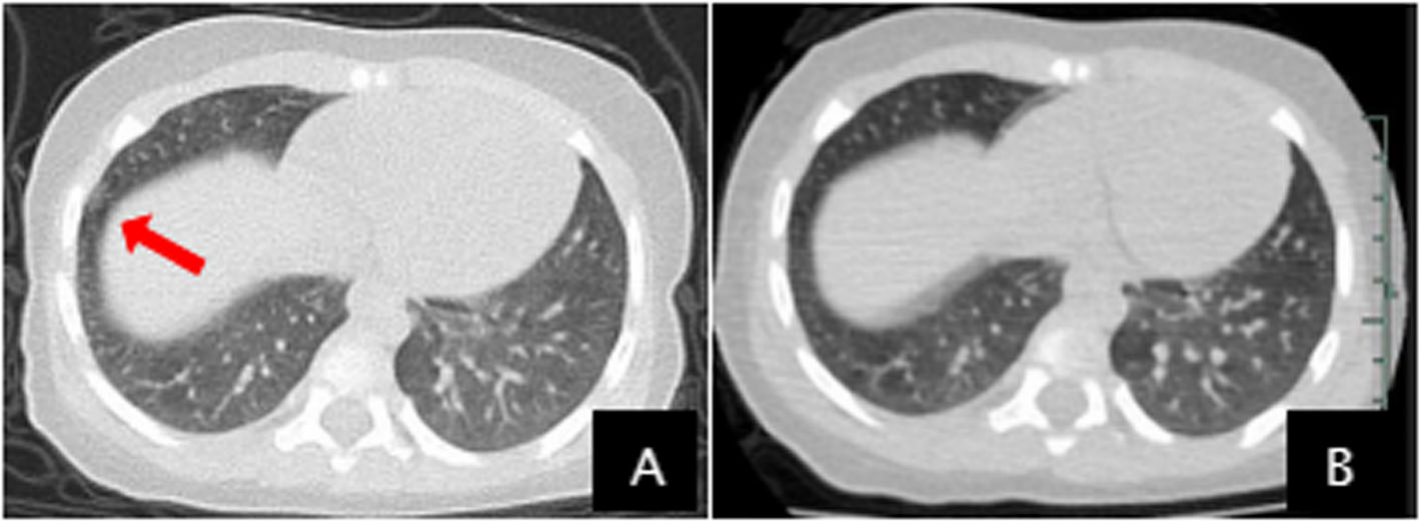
a-b: Chest CT plain scans of the child. a. Hospital day 4: ground-glass shadows in the lower lobe of the right lung; b. Hospital day 28: the ground-glass shadows in the lungs have basically absorbed
